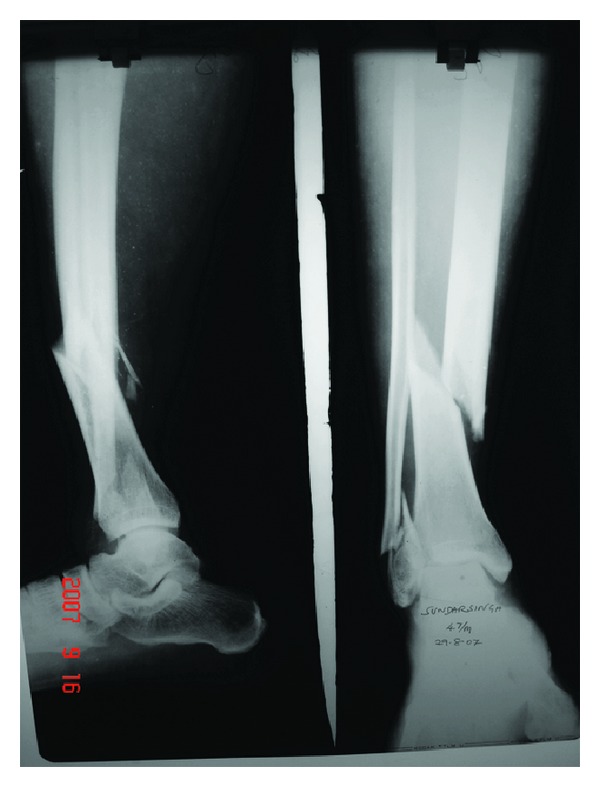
Preoperative X-ray showing varus and antecurvatum.
